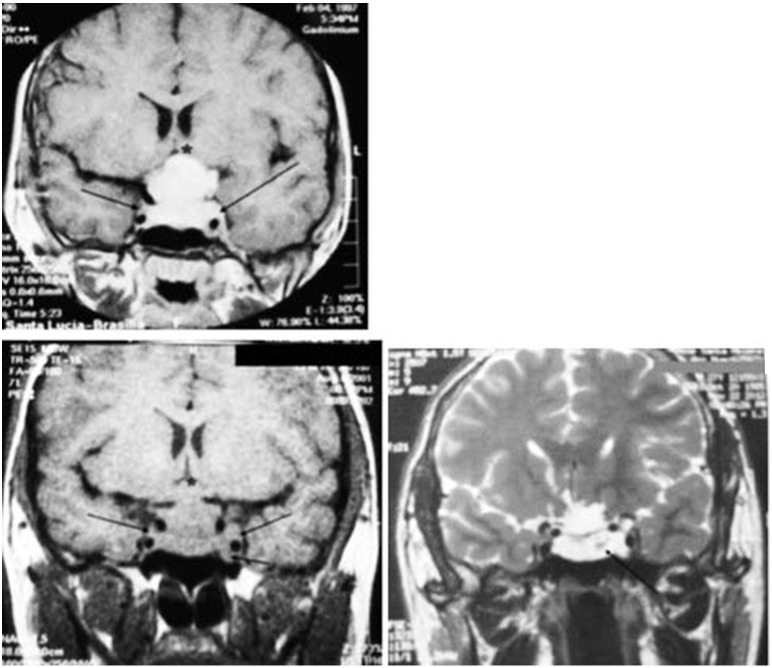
Magnetic resonance imaging of the sella turcica on T1 coronal, with homogeneous contrast, performed before transcranial surgery and the start of the treatment with bromocriptine, which shows the pituitary adenoma with invasion of the cavernous sinus on both sides (black arrows) and compression of the optic chiasm (^*^). Without contrast, performed 41 months after starting the treatment with dopamine agonists (34 months of using bromocriptine and 7 months of using cabergoline), showing the pituitary adenoma with invasion of the cavernous sinus on both sides (black arrows) and compression of the optic chiasm. On T2, 14 years after starting the treatment with dopamine agonists, there is a marked decrease in the size of the pituitary adenoma with formation of a cystic mass (black arrow).
